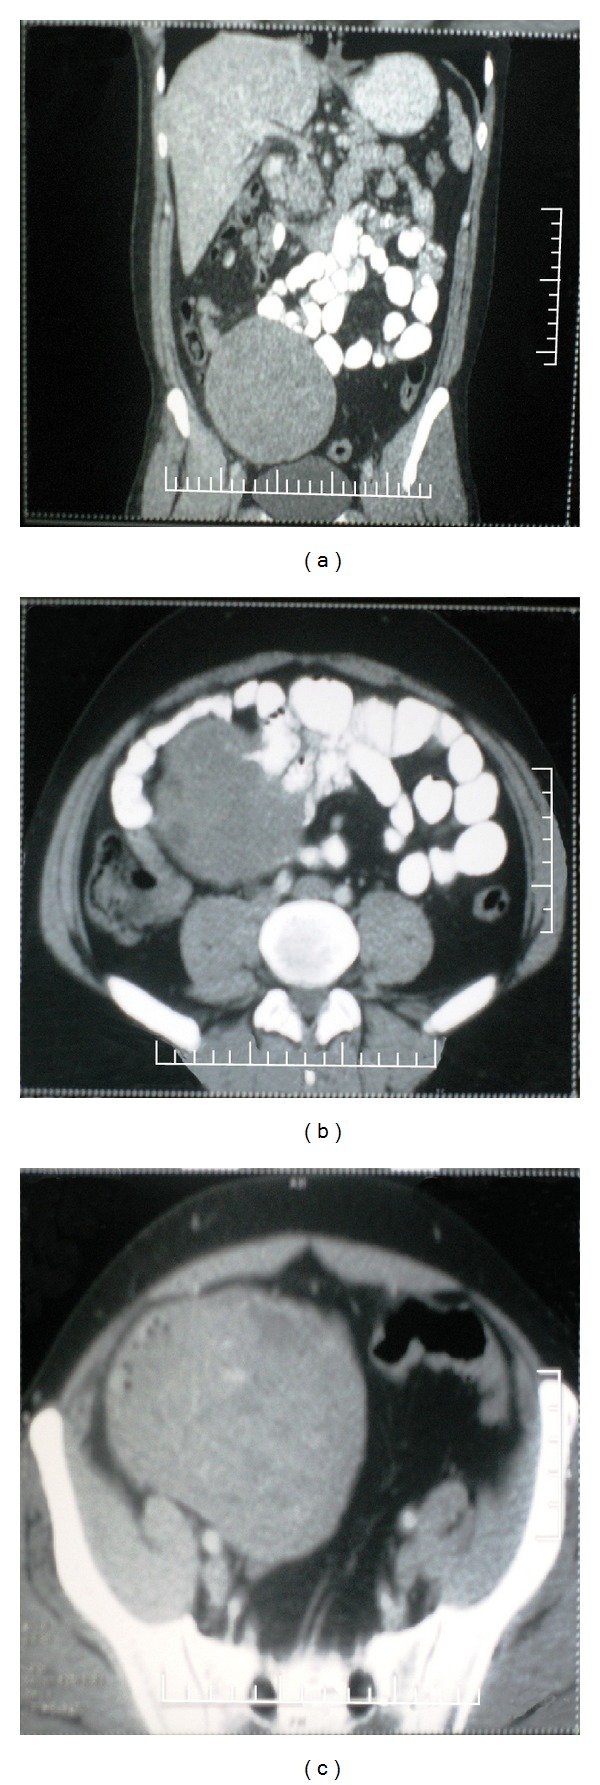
Computed tomography enteroclysis showing a large well-defined heterodense ovoid space occupying lesion measuring 15 × 10 cm with clumped bowel loops seen in right iliac fossa.
